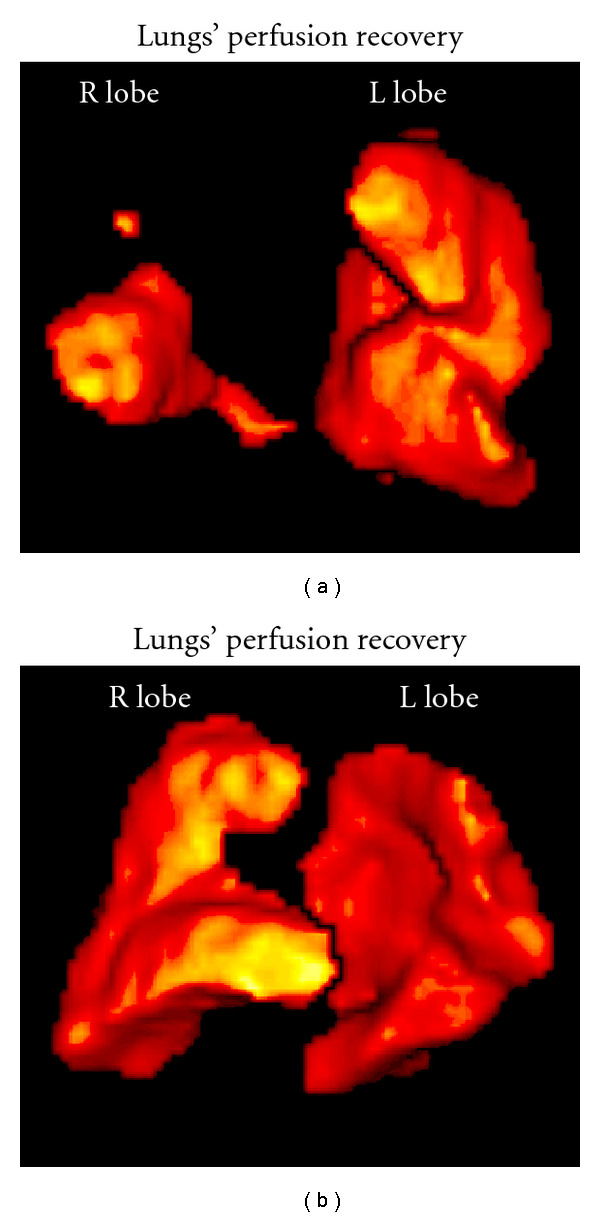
3D SPECT Lungs' FBP reconstruction by Hann filter (critical frequency 0.9) and Chang attenuation correction order 0, coefficient 0.11. (a) 4 hours postevent, Right lobe embolism, R lobe volume 0.66 lt, and total lungs' volume 2.85 lt. (b) 11 days posttherapy, R lobe volume 3.28 lt, and total lungs' volume 5.28 lt. [37] *(modified)*.
